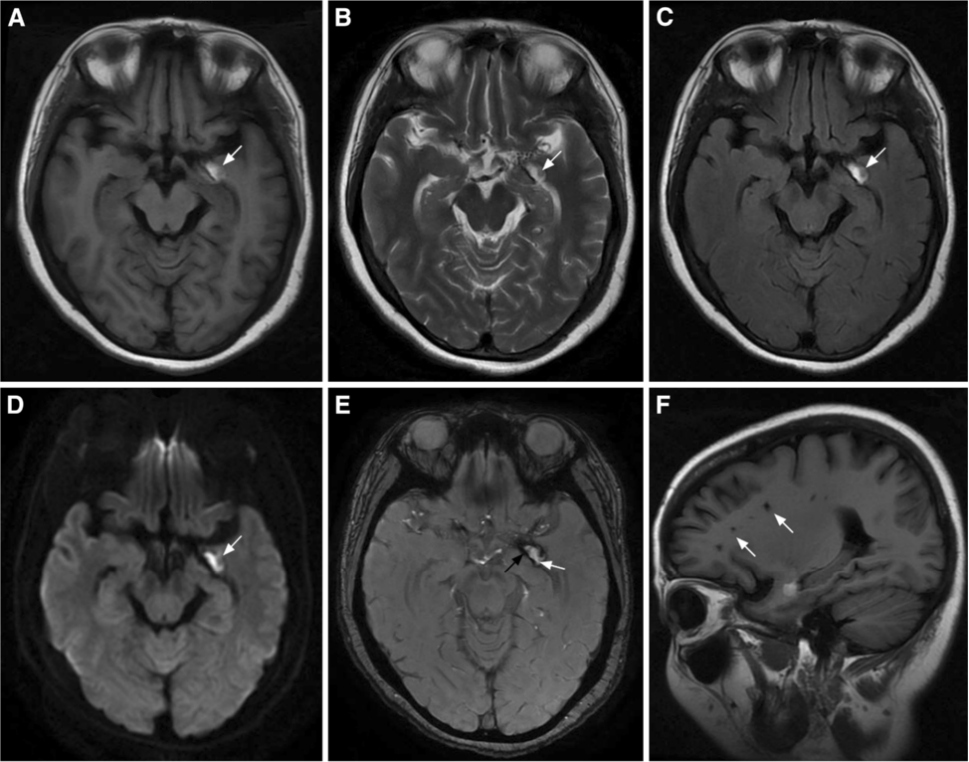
Brain MRI obtained 5 days post-onset. Axial T1, T2, T2-FLAIR, and DWI (A–D) show an abnormal high signal within the left lateral uncus (hippocampus) (arrows). On T2 star-weighted angiography imaging (E), the lesion shows markedly low signal intensity (black arrow) with a hyperintense core (white arrow). These findings indicate that the left hippocampal hemorrhage was subacute. Sagittal T1 (F) shows multiple lacunar low signals in the parietal and periventricular areas (arrows), suggesting multiple lacunar infarction in the cerebral hemispheres.
